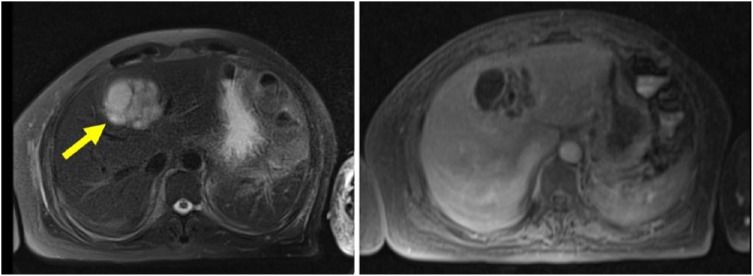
Liver magnetic resonance imaging demonstrating a pyogenic liver abscess. The arrow points to a thick-walled cystic lesion 6.3 × 4.6 cm with small amount of surrounding edema (the image on the left is a T1 image and right is a T2 image). There is no intrahepatic or extrahepatic biliary dilatation, lymphadenopathy, or free fluid. Aspiration of the complex multiloculated lesion returned purulent material consistent with pyogenic hepatic abscess.
